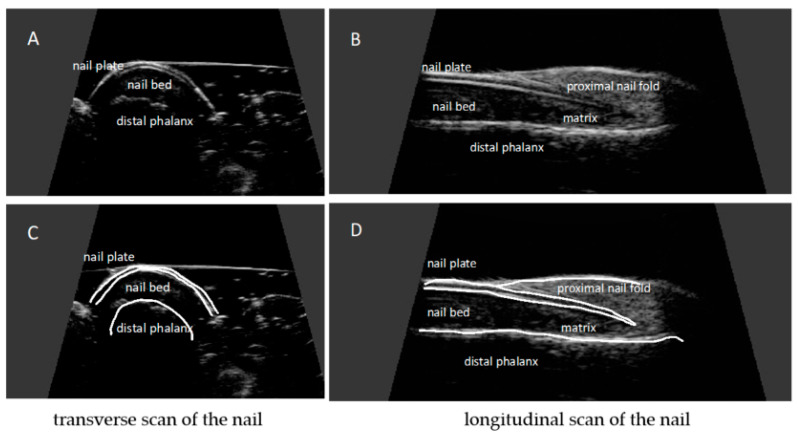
Ultrasonographic image of a healthy nail: transverse (A,C) and longitudinal view (B,D). Nail plates presented as a structure consisting of two parallel hyperechoic plates separated by a hypoechoic interlaminar space. Nail matrix presented as an isoechogenic structure in the proximal part of the nail. Nail bed presented as a hypoechoic structure between the ventral nail plate and the periosteum of the distal phalanx.
